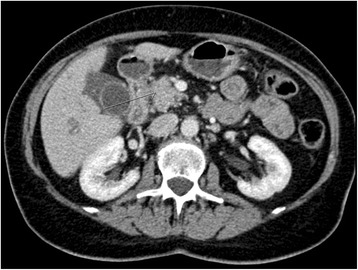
August 2008. Contrast-enhanced abdominal computed tomography shows a tumor in the head/uncinate process of the pancreas
